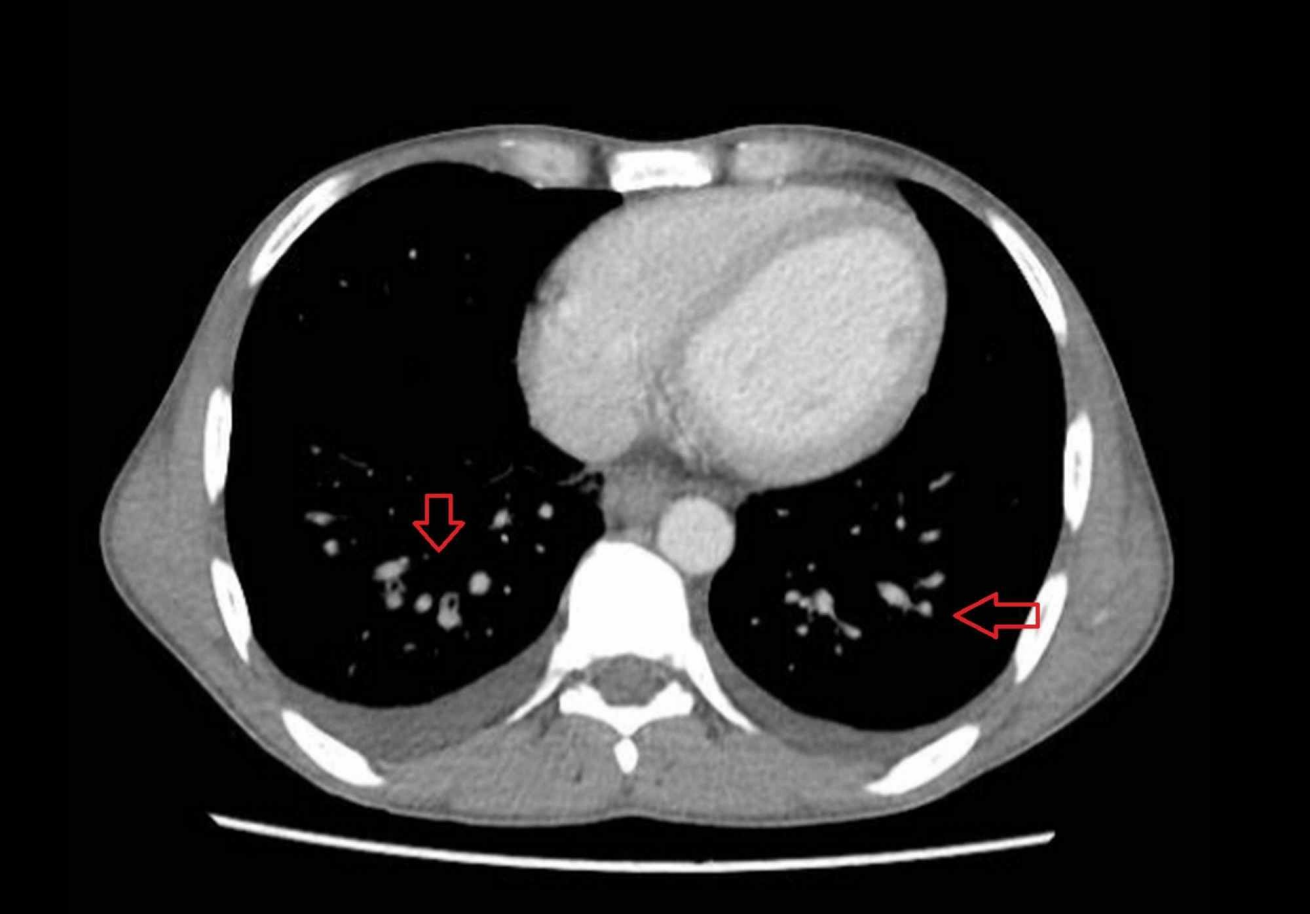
CT scan of chest showing variable-sized nodules (red arrows) in bilateral lung bases.
